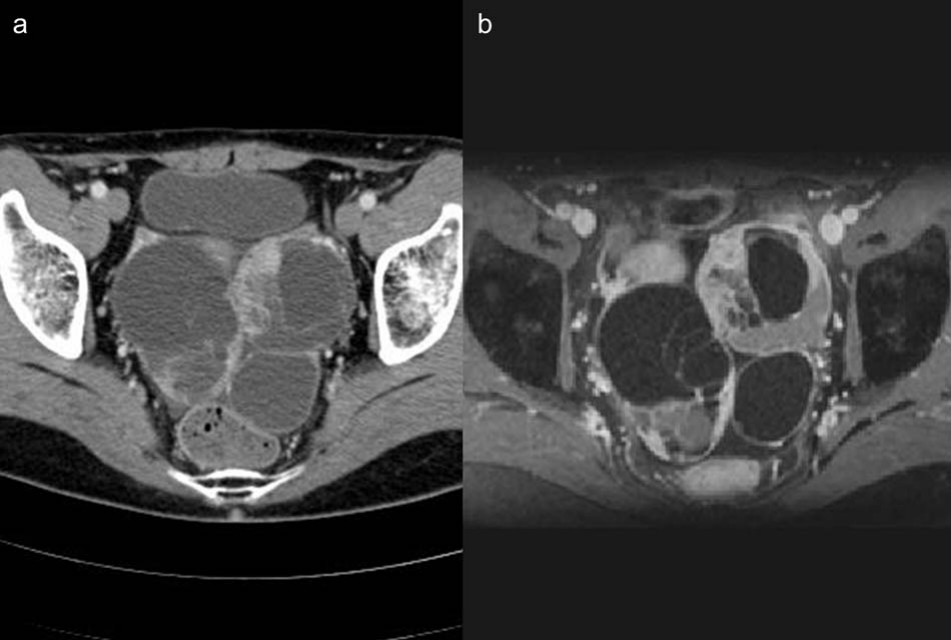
(a) Computed tomography scan showing a multi-cystic mass in the pelvis with a partial contrast effect. (b) Gadolinium-enhanced T2-weighted magnetic resonance image showing a solid mass with contrast effect in the cysts.
